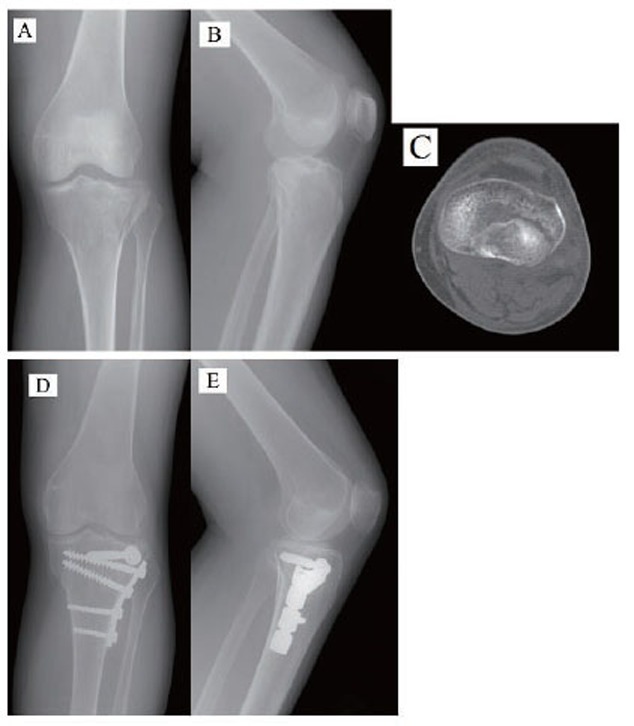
(A–C), Anteroposterior, lateral radiographs, and computed tomography scans of the right knee in a 43-year-old female showing the split-depression fracture of the posterolateral tibial plateau. (D, E), Radiographs showing the fracture reduction and implant position following surgery.
